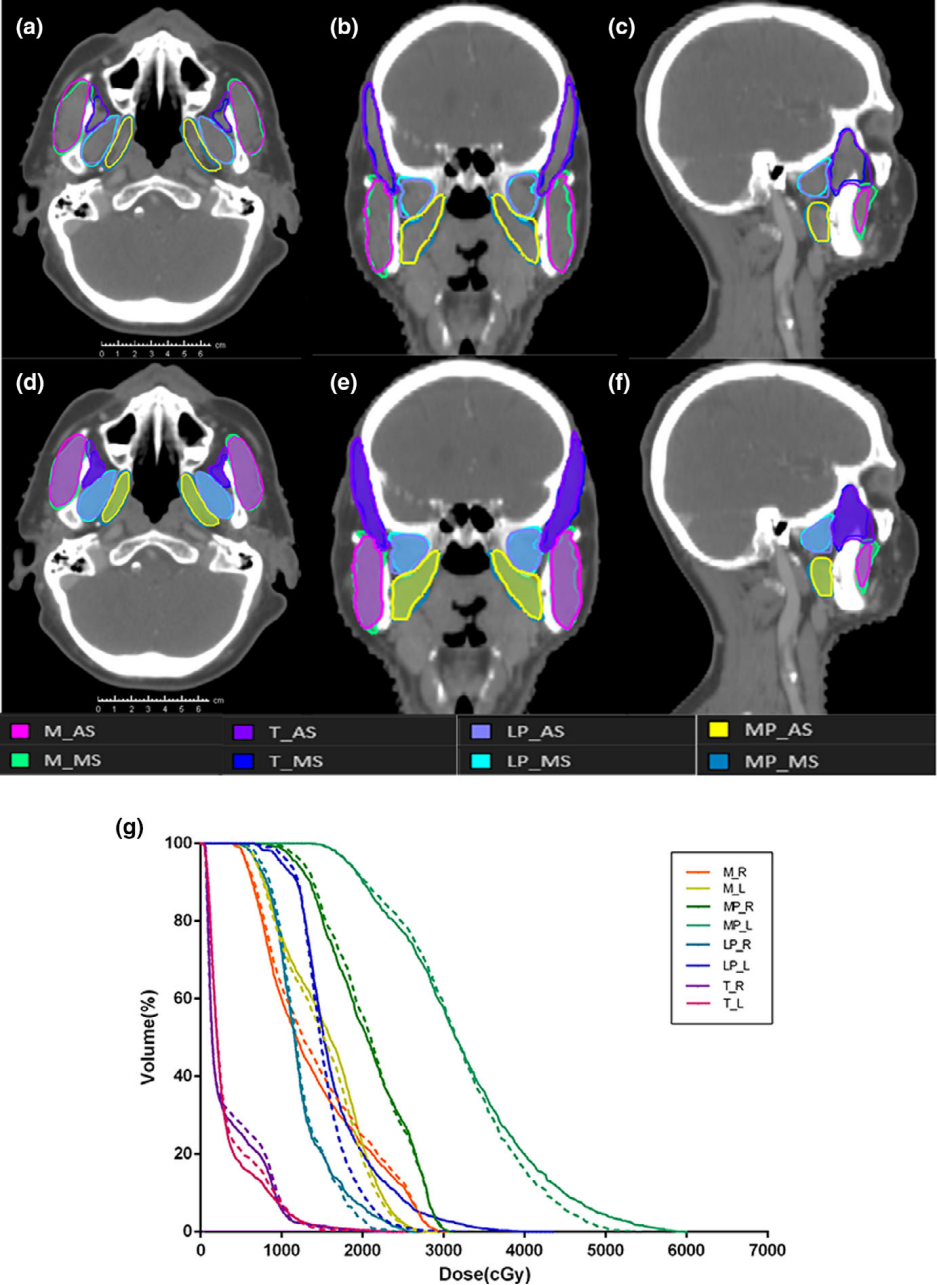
(a) Representative CT images of manual drawn and auto‐segmented contours for eight mastication muscles, respectively. A–C show manual contours (solid lines) and D–F show auto‐segmented contours (color wash). A and D: transverse section; B and E: coronal section; C and F: sagittal section. Masseter (M), temporalis (T), lateral pterygoid (LP), medial pterygoid (MP); (b) dose–volume histogram of eight mastication muscles for manual drawn (solid lines) and auto‐segmented (dashed lines) contours for the patient in Fig. 1. Masseter‐right (M‐R), masseter‐left (M‐L), temporalis‐right (T‐R), temporalis‐left (T‐L), lateral pterygoid‐right (LP‐R), lateral pterygoid‐left (LP‐L), medial pterygoid‐right (MP‐R), and medial pterygoid‐left (MP‐L).
